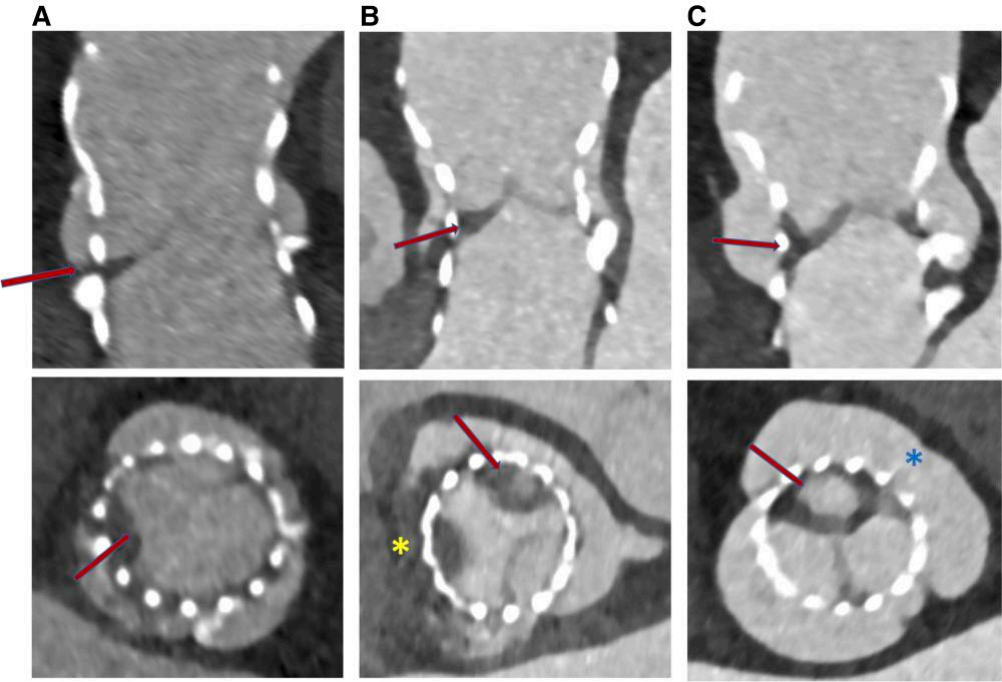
Example of hypoattenuated leaflet thickening visualised with multi-detector computed tomography in the long and short axes (red arrows); (*A*) hypoattenuated leaflet thickening < 25%; (*B*) hypoattenuated leaflet thickening 25–50%; (*C*) hypoattenuated leaflet thickening > 75%. Asterisk in the (*B*) panel refers to association with sinus thrombosis; asterisk in the (*C*) panel refers to bicuspid valve.
